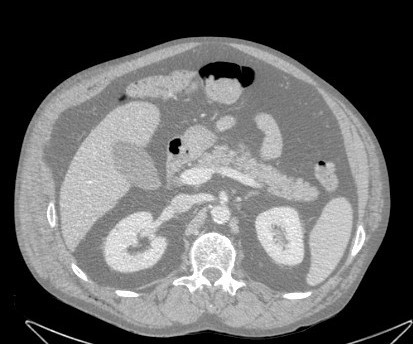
CT scan on admission. No intestinal herniation.
